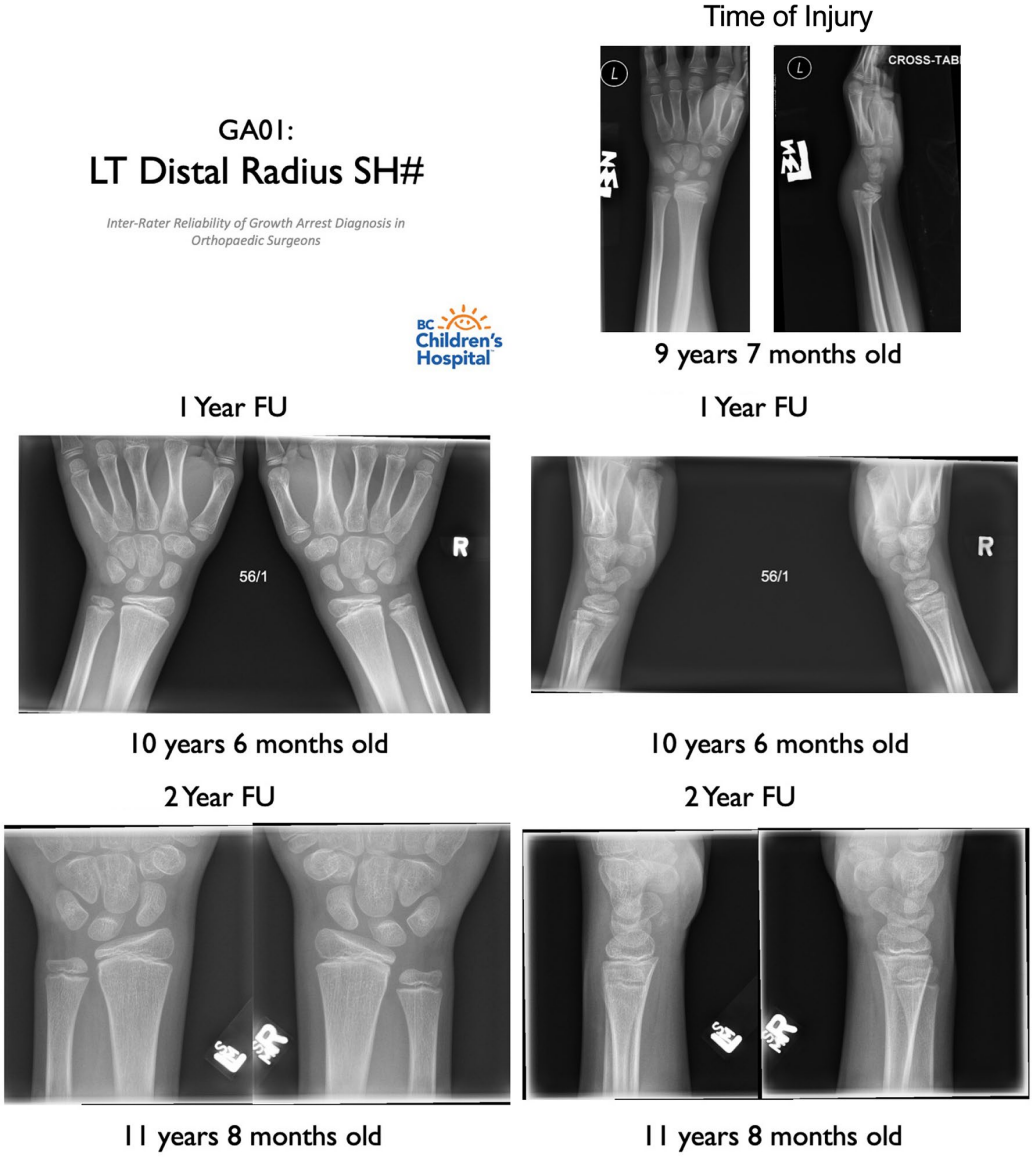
Example of a patient case containing anteroposterior (AP) and lateral radiographs from immediate post-injury and long-term follow-up visits in a patient with a physeal fracture. Each patient case specified the side and anatomical site of the physeal fracture. Immediate post-injury radiographs of the injured side were presented with the date of injury and the patient’s age at injury. Long-term follow-up (one- or two-year) radiographs of the injured and contralateral sides were presented with the date of follow-up and the patient’s age at follow-up. Surgeons were asked whether or not they would diagnose the patient with growth arrest based on the radiographs provided by answering ‘yes’ or ‘no’ on a data collection form
